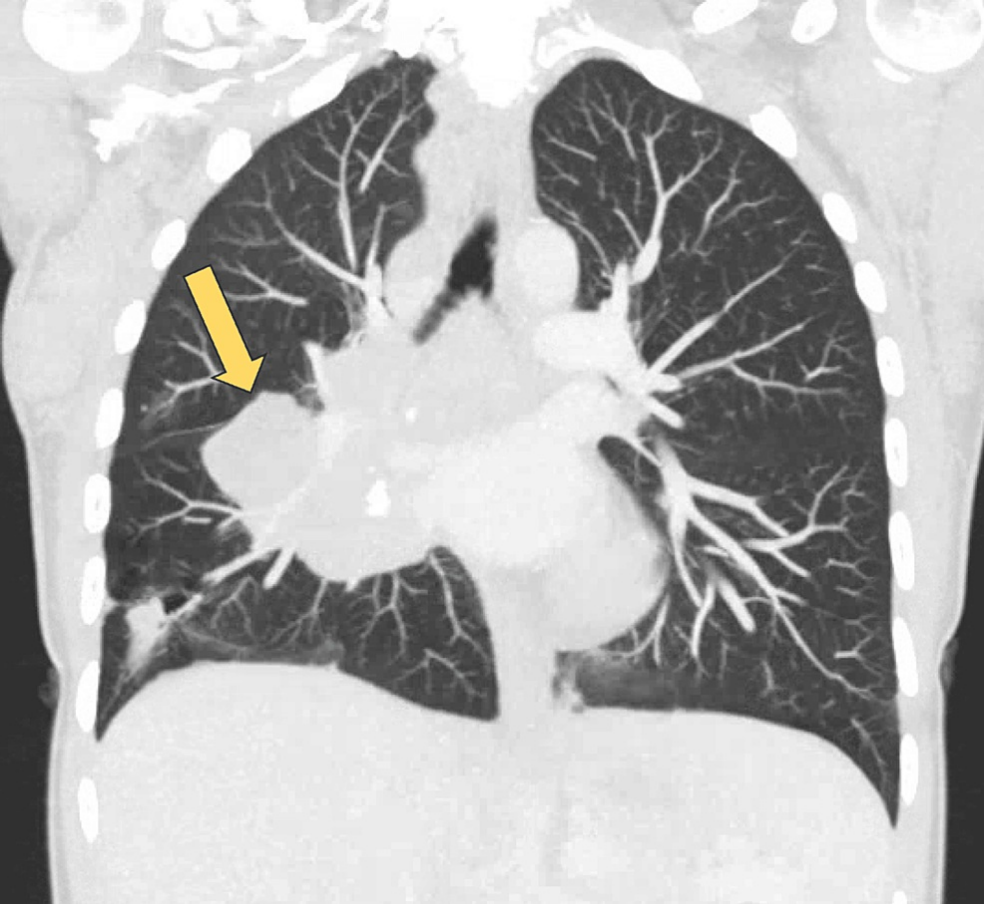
Coronal computed tomography image of the chest with contrast showing bulky right paratracheal and hilar lymphadenopathy and a right lower lobe mass.
